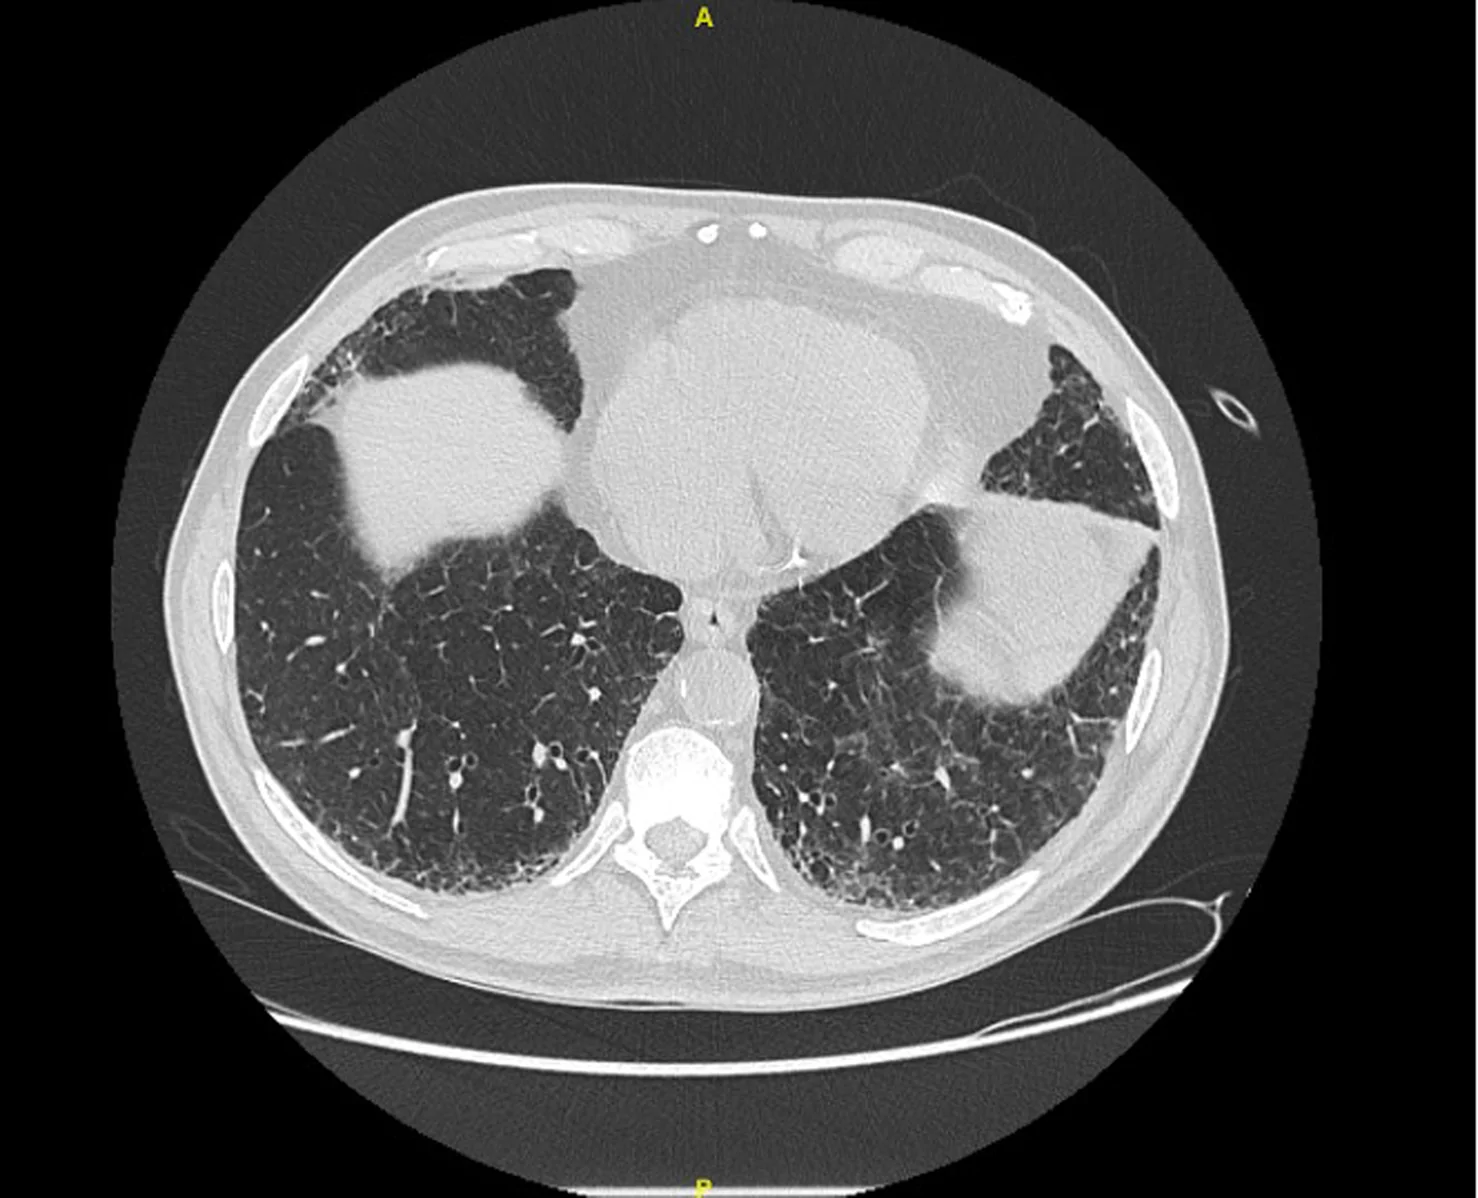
CT chest imaging demonstrating subpleural reticulation, traction bronchiectasis, and bronchiolectasis with basal predominance, stable from prior imaging, meeting criteria for probable usual interstitial pneumonia.^5^
